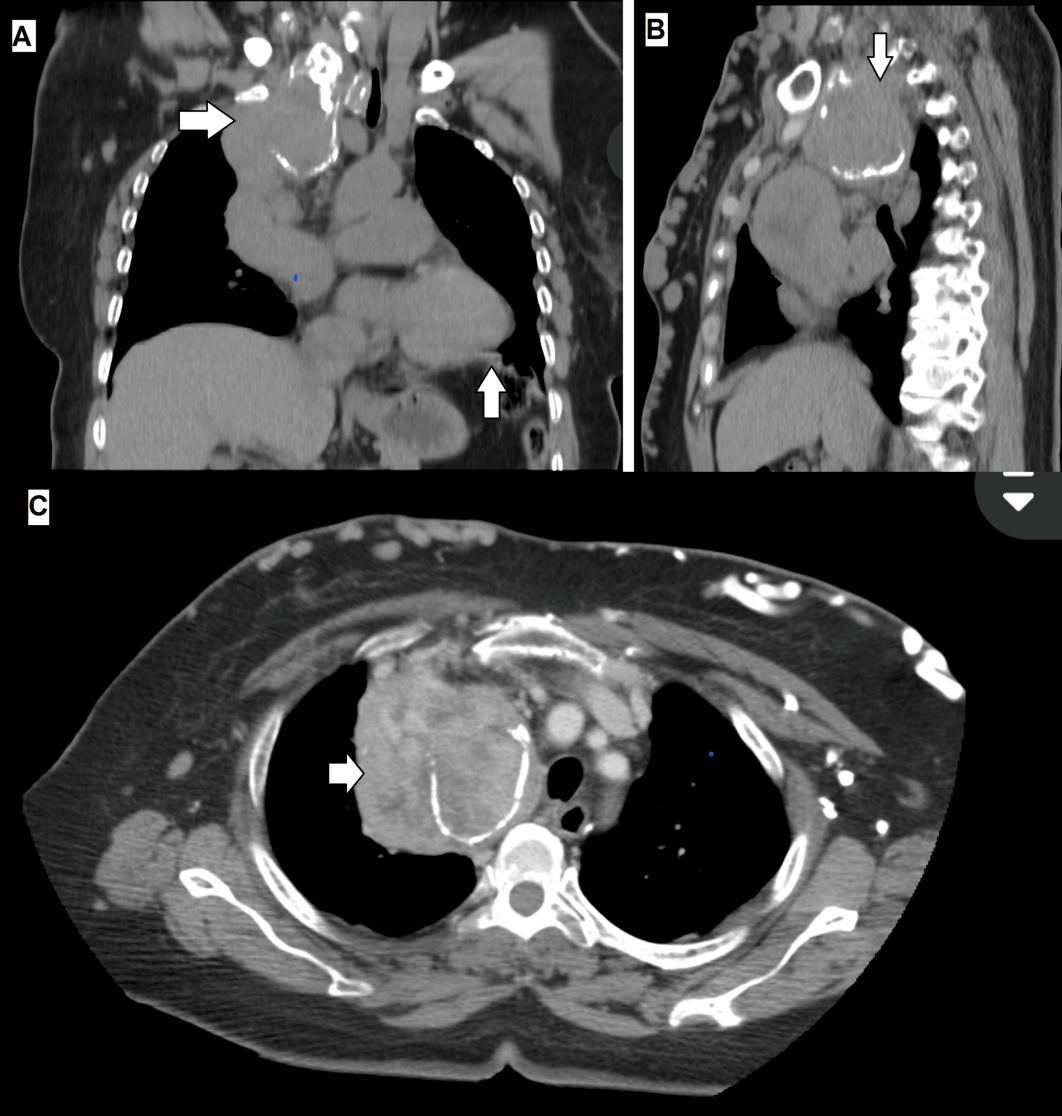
Contrast-enhanced chest CT (A) Coronal view showing a bilobulated anterior mediastinal mass compressing the right lung apex. (B) Sagittal view demonstrating severe compression of the superior vena cava and brachiocephalic trunk. (C) Axial view highlighting the mediastinal mass with heterogeneous density and wall calcifications, causing marked collateral venous dilatation.
